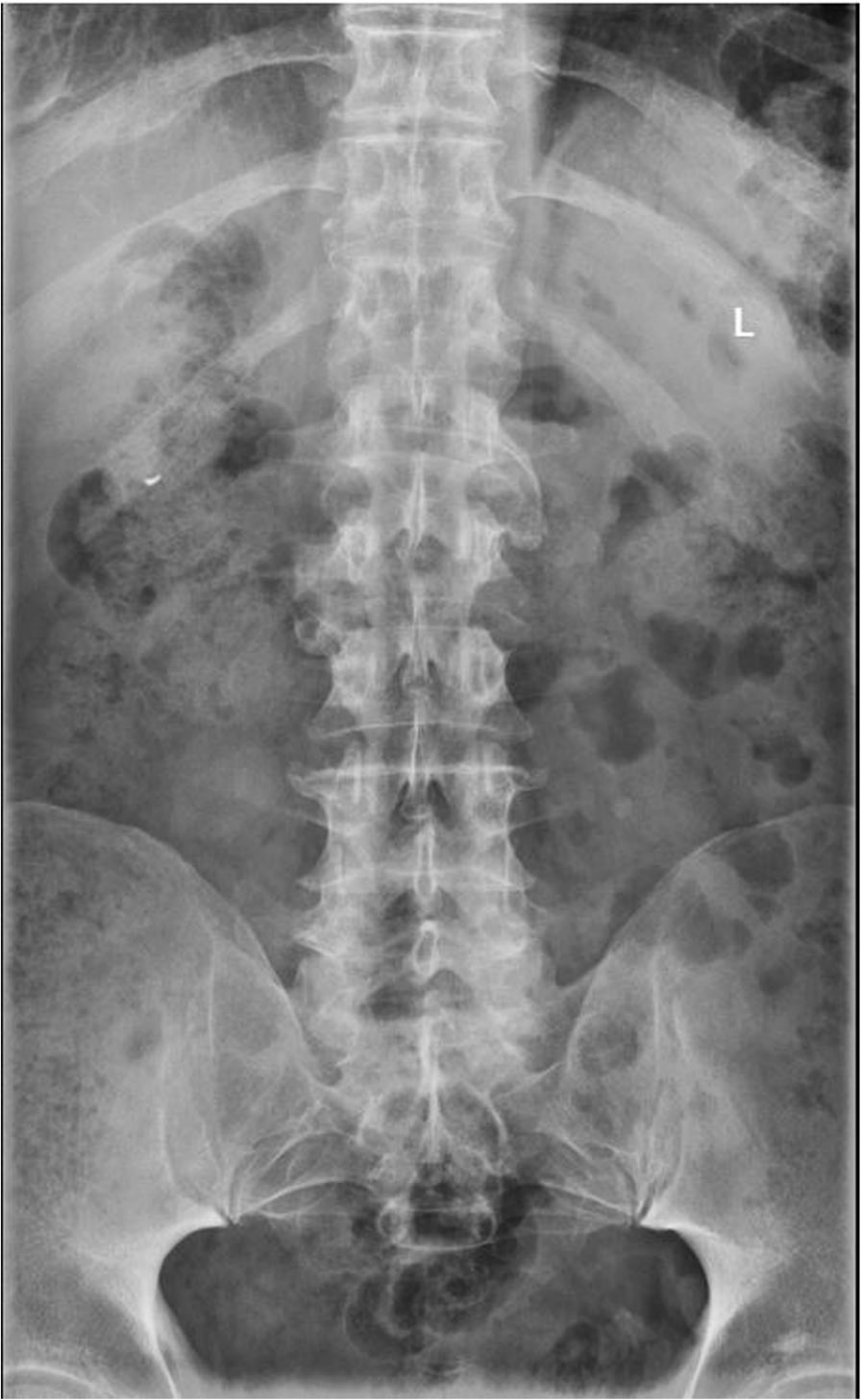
AP Lumbar Plain Films; preserved sacroiliac joint lines
